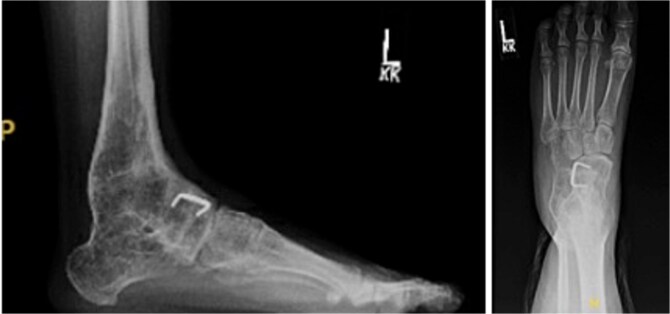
Postoperative radiographs of the right ankle following hardware removal and anterior tibial exostectomy. The lateral and dorsoplantar views demonstrate removal of prior fixation with maintained alignment and evidence of a healed ankle fusion.
